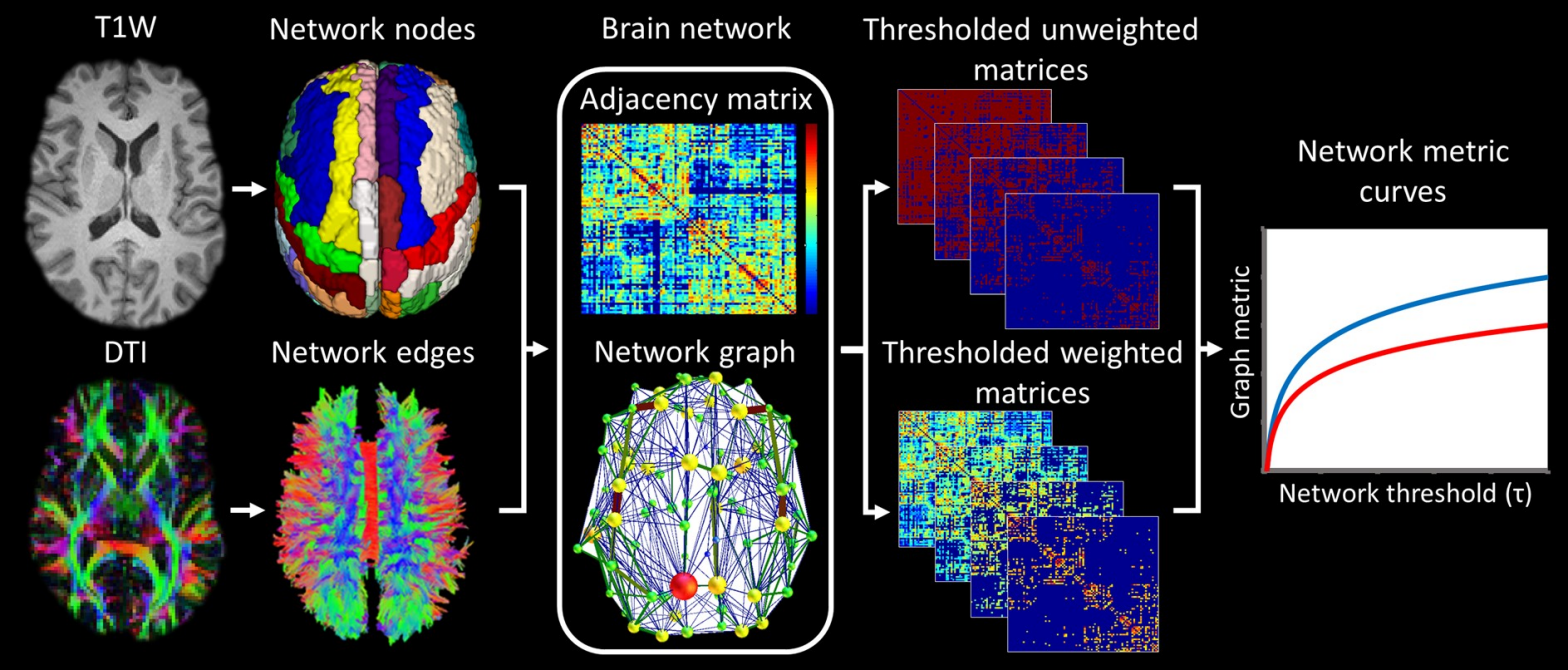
Network construction. The native T1-weighted images were co-registered to the DTI and transformed to Montreal Nerological Institute space. These transforms were combined, inverted and applied to the AAL atlas (excluding cerebellum) parcellating 90 anatomical regions on the DTI (Network nodes). White matter fibre tracts were traced from the DTI (Network edges). Structural networks were defined from the nodes and edges, and the edges weighted by the number of constituent streamlines adjusted for streamline length and end-node volume. Networks were thresholded across 40 edge weighting and edge density thresholds. Weighted and unweighted network metrics were calculated at each threshold and used to construct network metric curves.
